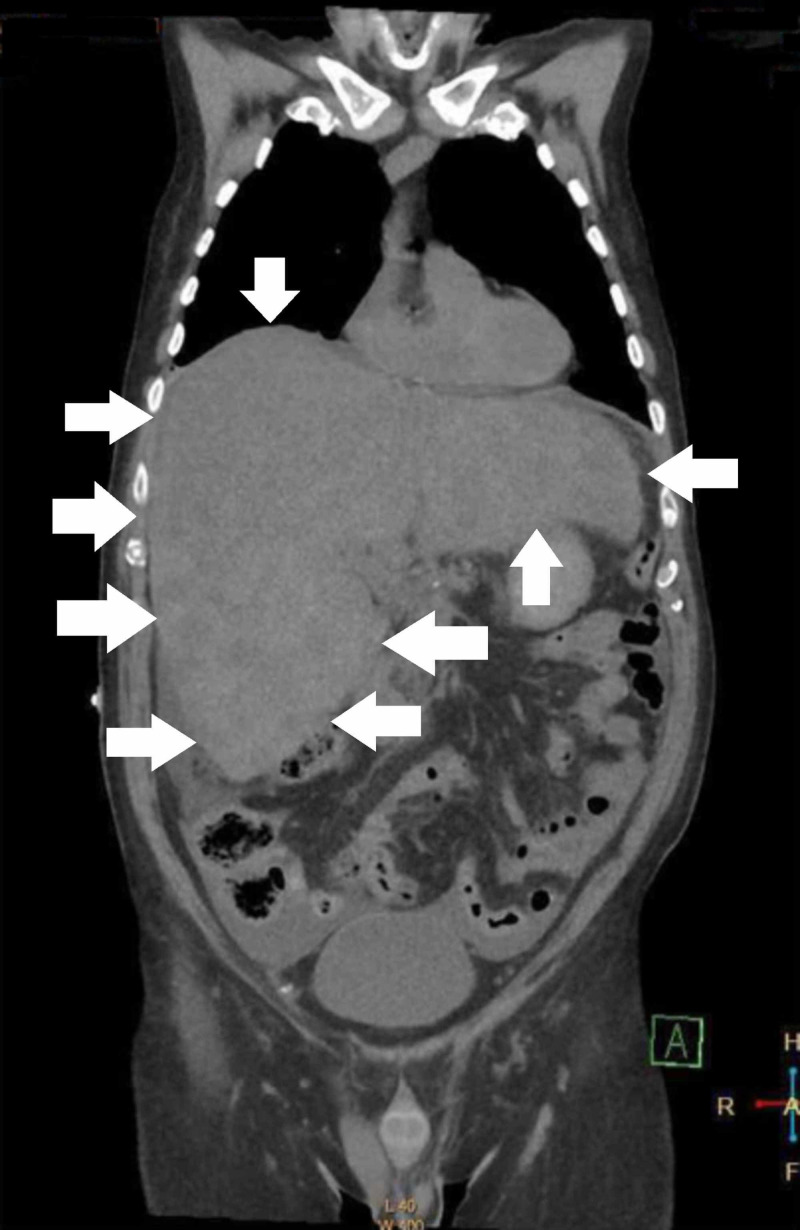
CT of the chest, abdomen, and pelvis, showing a coronal view of the grossly enlarged liver
